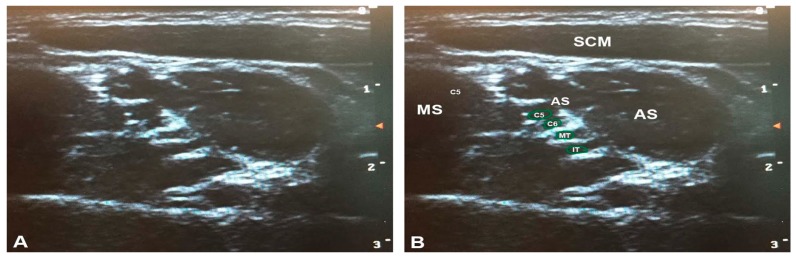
The C5 piercing variant, identified using ultrasonography in the present study: (A) unlabeled; and (B) labeled. Note that the C5 anterior ramus is not separated from the anterior scalene in this condition, visible as a lack of hyperechoic fascia. AS =anterior scalene; C5 = anterior ramus of C5; C6 = anterior ramus of C6; IT = inferior trunk; MS = middle scalene; MT = middle trunk; SCM = sternocleidomastoid; ST = superior trunk. The green outlines demarcate the trunks and roots of the brachial plexus.
